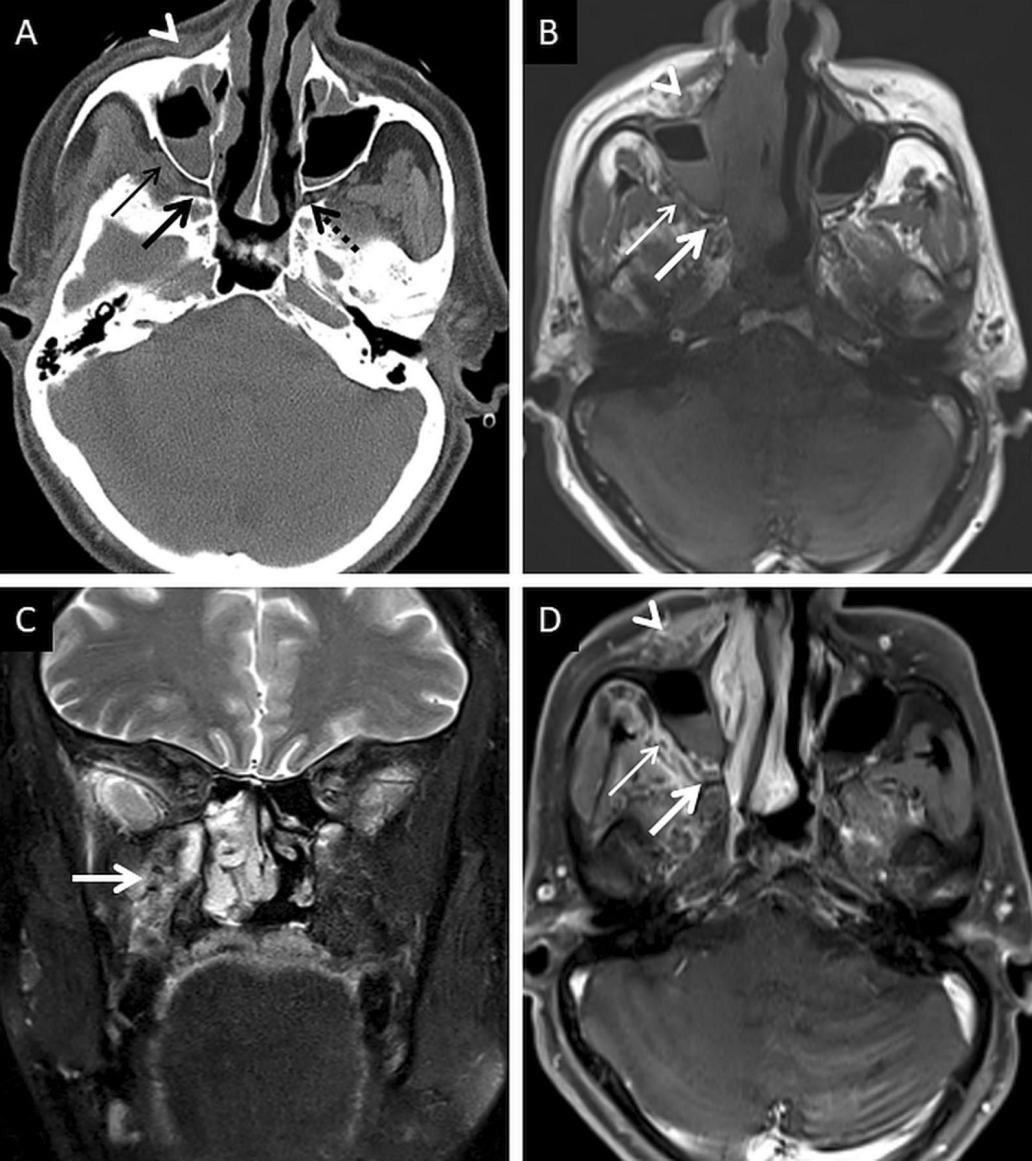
PPF involvement in ROCM. Unenhanced axial CT scan of the brain (a) reveals soft tissue within the right PPF (thick arrow). Note the normal fat density and branches of the internal maxillary artery seen in the contralateral, uninvolved PPF (dotted arrow). Soft tissue is also noted in the right retro-antral fat (thin arrow) and the right anterior periantral fat (arrow head) as well. Based on the imaging signs, the possibility of ROCM was suggested and a prompt CE MRI of the neck was performed. Unenhanced axial T1W (b) and coronal T2-FS (c) images of the neck confirm the presence of soft tissue in the right PPF (thick arrows in b, c), right retro-antral fat (thin arrow in b) and right anterior periantral fat (arrow head in b). CE FS axial T1W image (d) reveals heterogeneous enhancement of the soft tissue in the right PPF (thick arrow) as well as in the right retro-antral fat (thin arrow) and right anterior periantral fat (arrow head)
